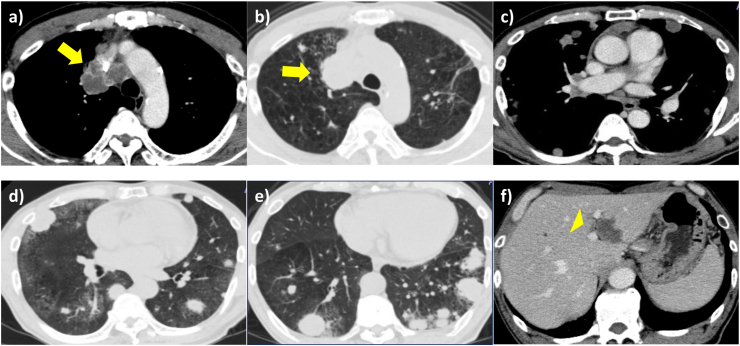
Chest computed tomography obtained on admission showing a-e) a right upper lobe tumor with enlarged mediastinal lymph nodes (arrow), tumors in the bilateral lung fields and f) a low-density area within the liver (arrowhead).
